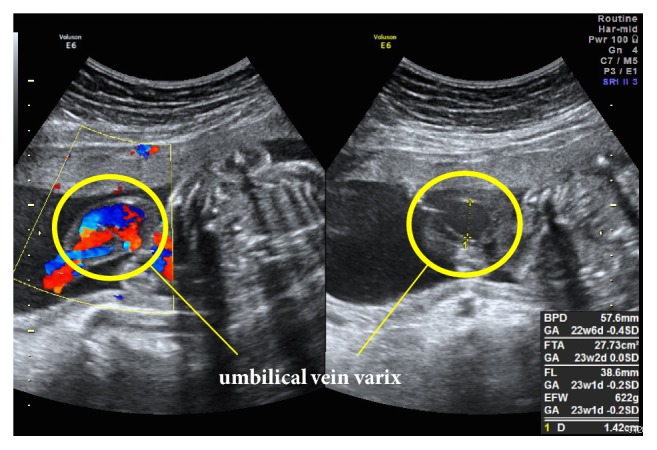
Ultrasonogram at 23 weeks of gestation shows abnormal bean-like dilation of the umbilical vein.
